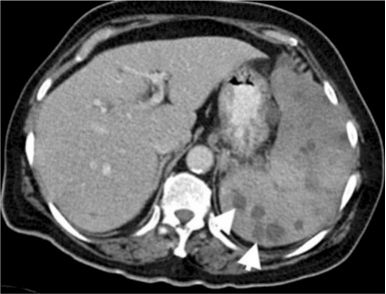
Splenic infection. Axial contrast-enhanced CT of a 35-year-old man with acute myeloblastic leukemia shows low-attenuated multiple focal lesions (*arrowheads*) representing splenic candidiasis
